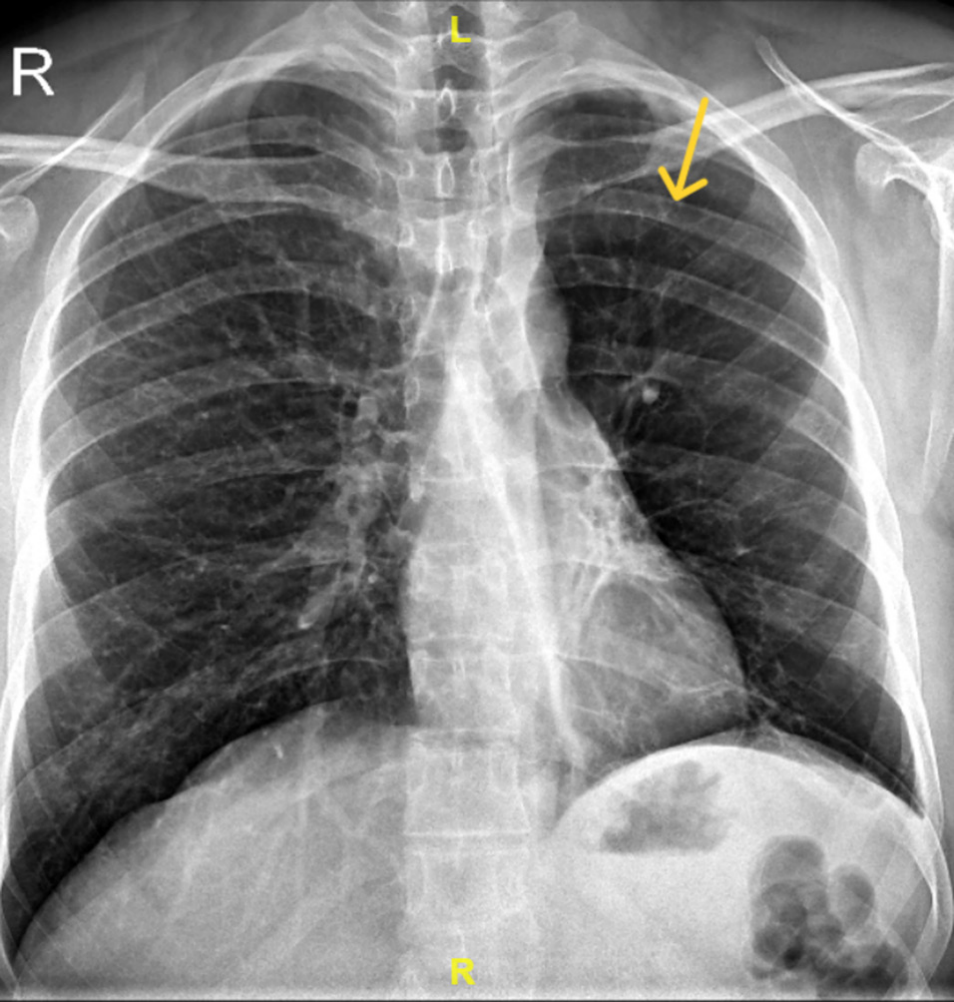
Plain chest radiography (X‐Ray) showing a hyperlucent left lung.
